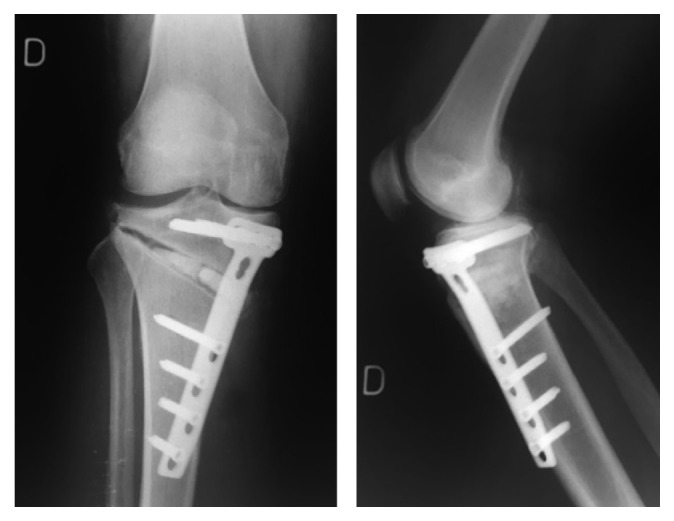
June 2009, 3 months after HTO, postoperative X-rays showing a slight pullout of the proximal screws. No fracture of the plate. No translation of the proximal fragment of the HTO.
